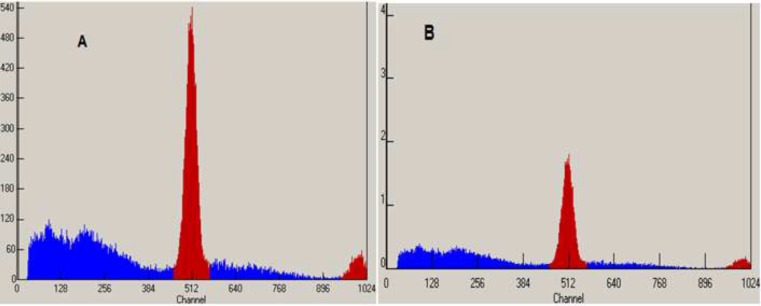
MCA Chromatograms of ^18^F-FDG after synthesis (A) and 10h later (B) at 35 - 40 ºC
